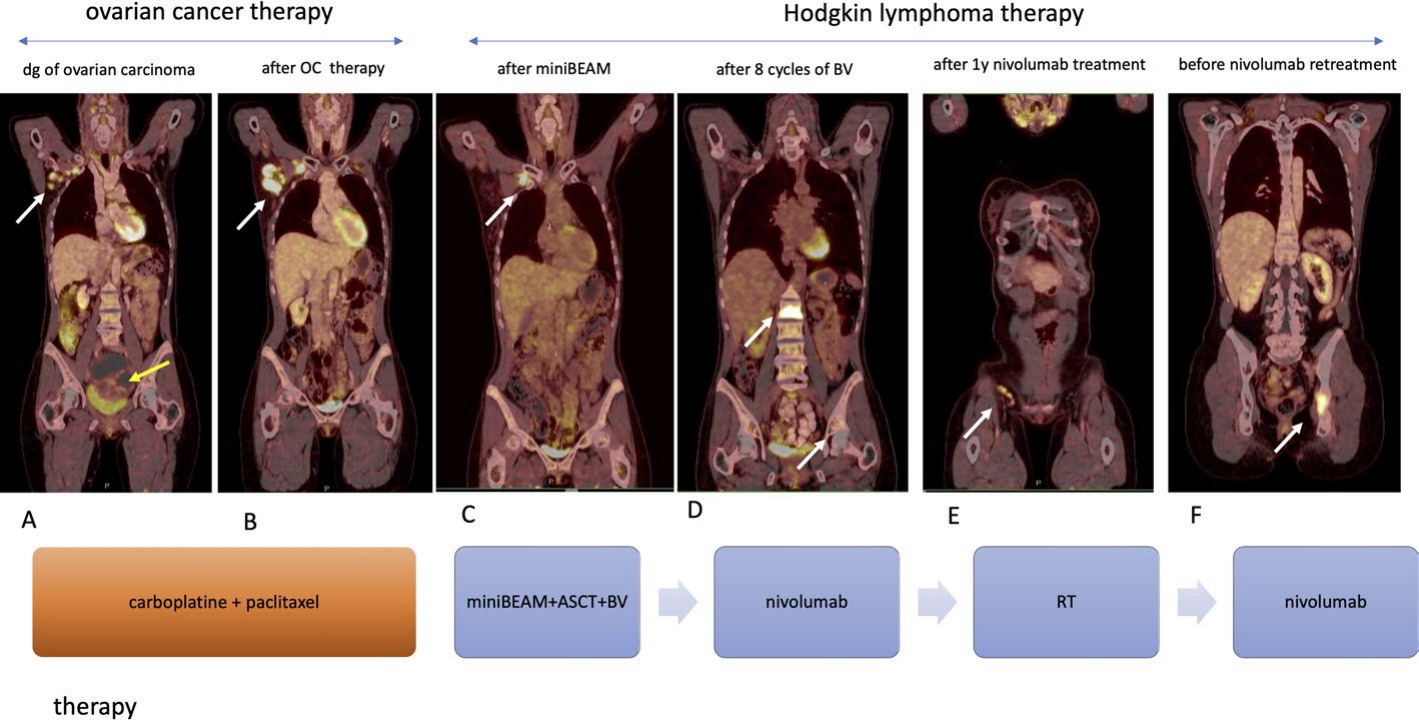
PET/CT findings during the treatment. White arrows—HL, yellow arrow—OC. A PET/CT scan at the time of diagnosis of ovarian carcinoma showing metabolically active axillar lymph nodes and heterogenous pelvic mass. B PET/CT scan after treatment of OC and before therapy of Hodgkin lymphoma showing complete regression of the pelvic mass and progression of axillar lymphadenopathy. C PET/CT scan after 2 cycles of chemotheraphy miniBEAM with ASCT D PET/CT scan after eight cycles of brentuximab-vedotin detected high metabolic activity in new locations, showing evident progression of the disease. E PET/CT scan after one year of treatment with nivolumab, with minimal residual involvement of inguinal lymph node. F PET/CT scan showing hypermetabolic lesion in left hip, before nivolumab retreatment
